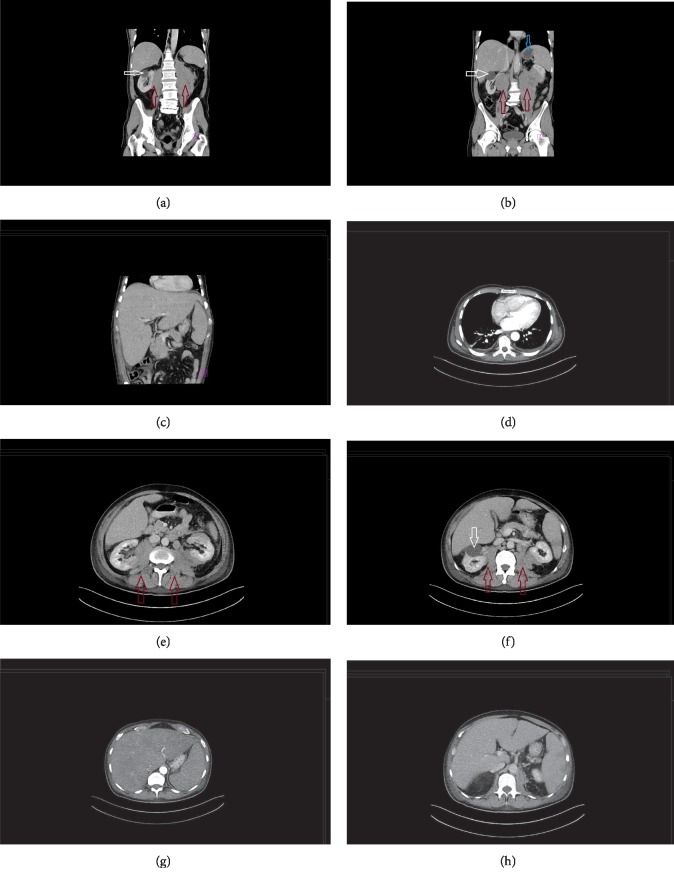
Computed tomography (CT) enhanced with the oral intake of diatriazoate meglumine and diatrizoate sodium contrast solutions. CT scans visualised a retroperitoneal mass of undetermined characteristics with infiltration of both iliopsoas muscles and kidneys, as shown both on sagittal and coronal views (marked with a red arrow in figures (a), (b), (e), and (f)). In the right kidney, a cyst measuring 4.5 cm was seen (marked with a white arrow in sagittal and coronal views in figures (a), (b), and (f)). An infarction zone in the spleen is shown in coronal view (marked with a blue arrow in figure (b)). There were no signs of bile duct dilation (as seen on figures (g) and (h) in sagittal view and (c) in coronal view). Sagittal view of the thorax did not show pathological changes (d). There were no specific signs of obstruction in renal system due to retroperitoneal mass, as well as no infiltration of liver and spleen (figures (a)–(c), and (e)–(h)).
